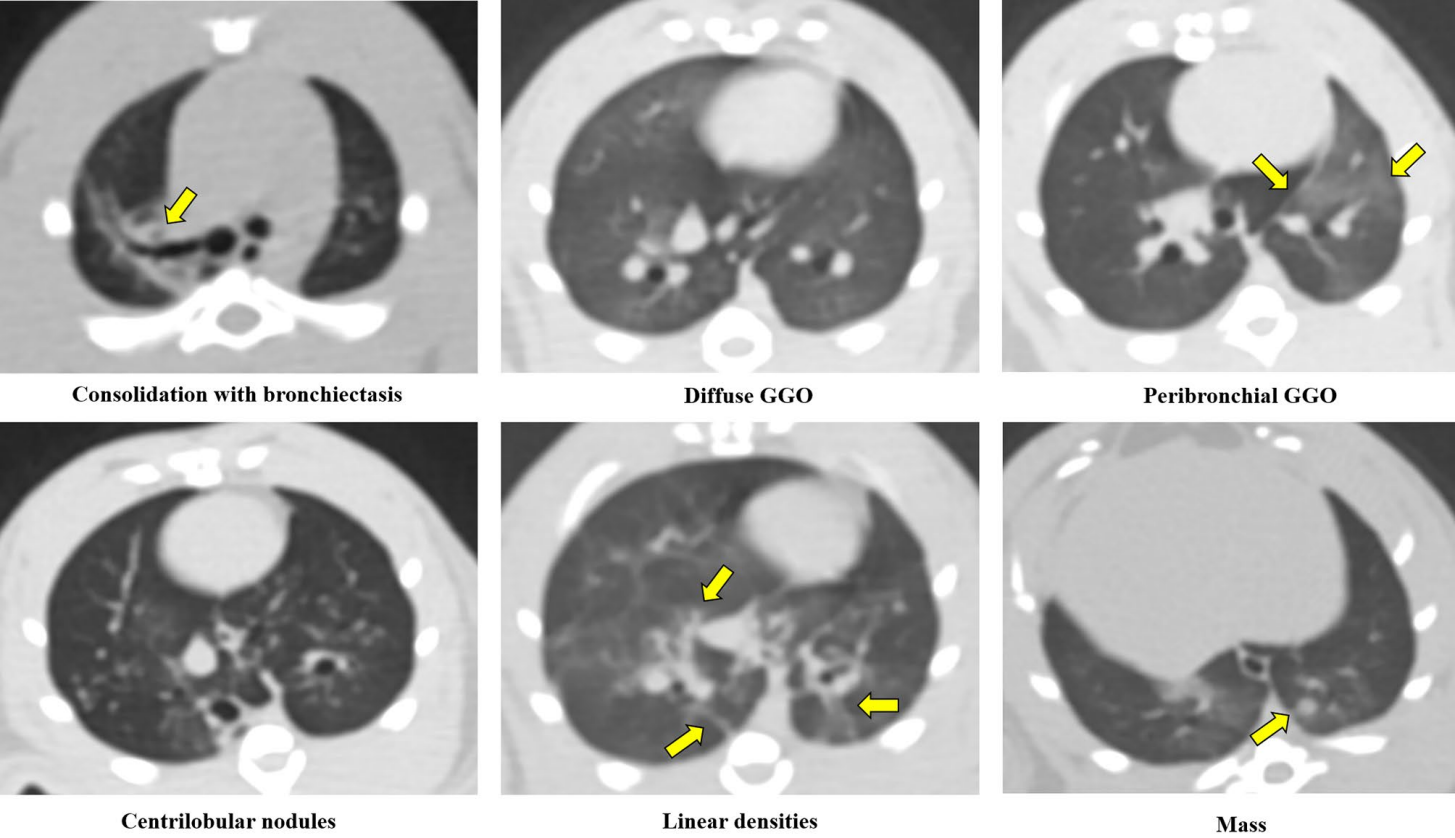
Examples of CT findings. Consolidation, ground-glass opacity (GGO), nodules, masses, centrilobular nodules, bronchiectasis, and linear atelectasis were followed or modified the glossary of radiologic terms suggested by the Fleishner Society.
